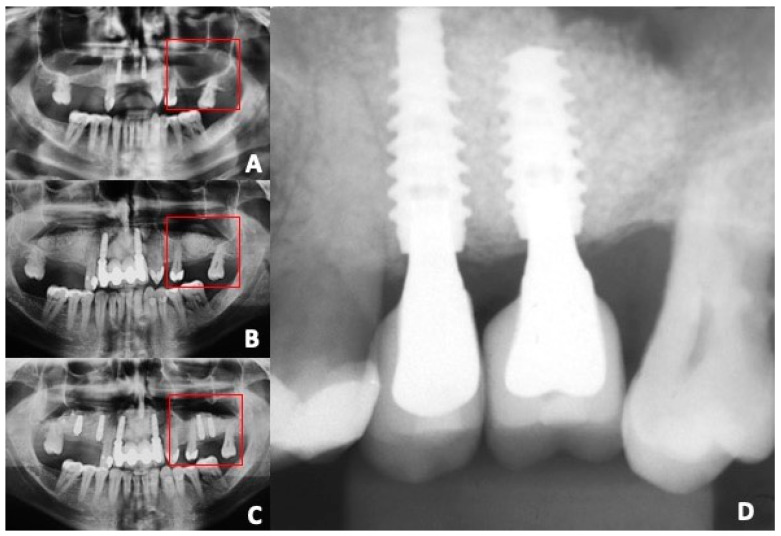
Radiographic view of the different stages of the maxillary sinus augmentation: (A) extensive pneumatization of the left side of the posterior maxilla; (B) 6 months after maxillary sinus augmentation with biphasic material showing a sufficient amount of newly formed bone; (C) 3 months after implant placement in the second premolar and first molar in newly formed bone after bone regeneration; (D) intra-oral radiographic image after 1 y loading showing no bone loss resorption around the implant–abutment connection. Red squares depicted the maxillary sinus augmented area.
